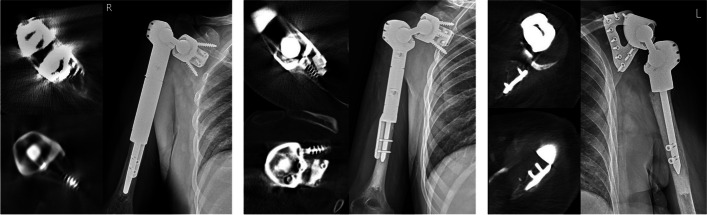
Final follow-up CT scans and radiographs. Imaging studies from all three patients at the time of last follow-up clinical visit (both CT and X-ray) demonstrating no signs of loosening of the glenoid component
